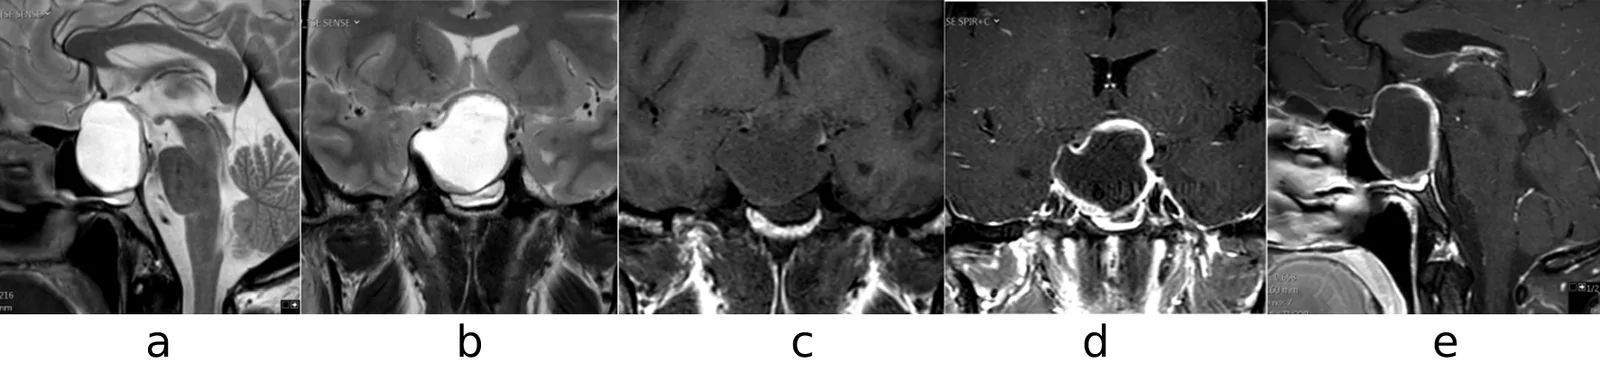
The case of a 43-year-old male with a surgically verified cystic pituitary adenoma. The sagittal and coronal T2-weighted images (a, b) and coronal T1-weighted image (c) show an intrasellar cystic mass extending into the suprasellar region. The cystic lesion reveals a thick wall on the contrast-enhanced coronal and sagittal T1-weighted images (d, e), which indicates a cystic adenoma of the pituitary rather than an RCC
